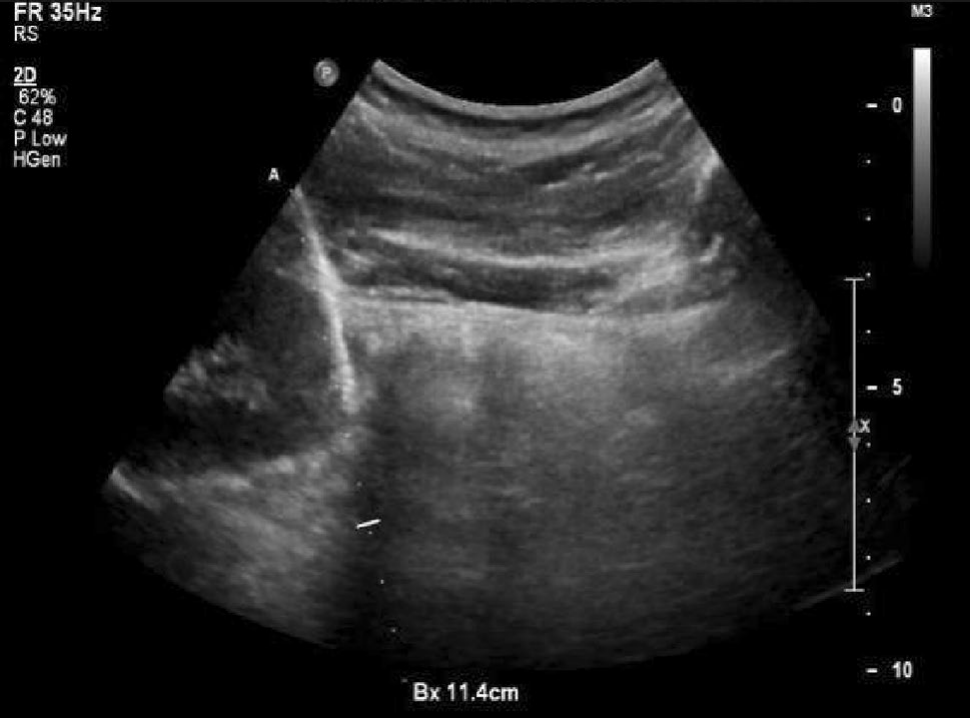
Ultrasound-guided line and puncturing route of kidney
